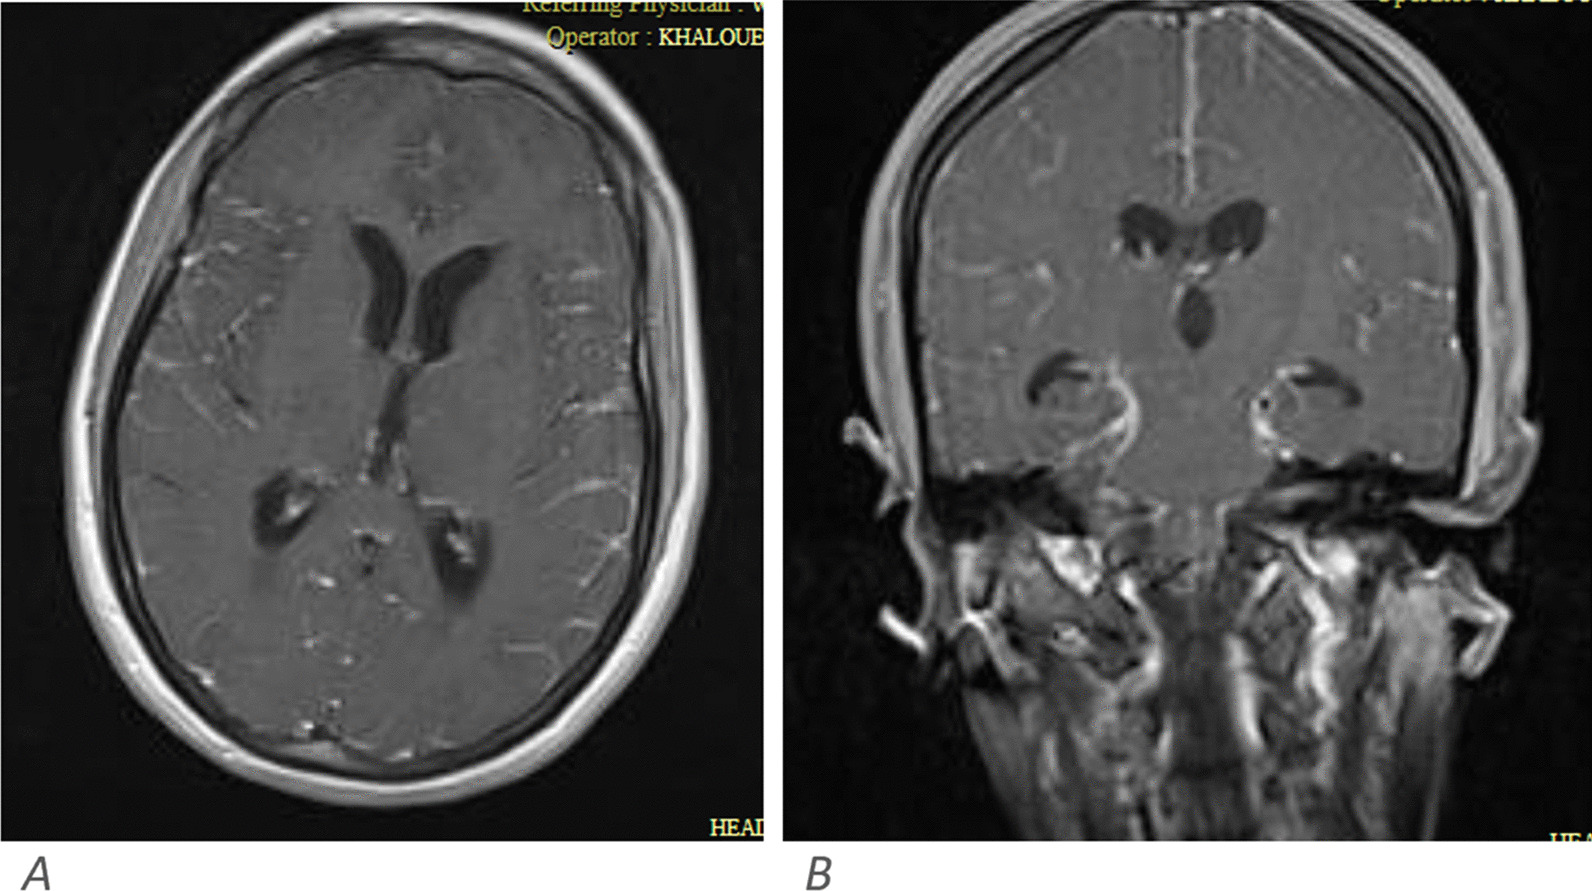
Axial (A) and coronal (B) post contrast T1-weighted images show diffuse leptomeningeal enhancement
